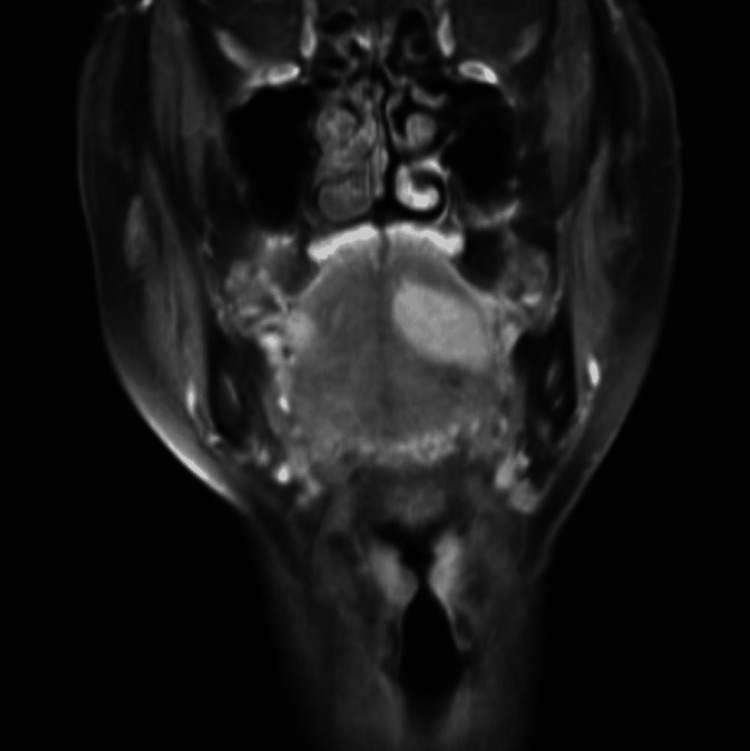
Contrast-enhanced T1-weighted coronal image with fat suppression showing a uniform contrast effect.
